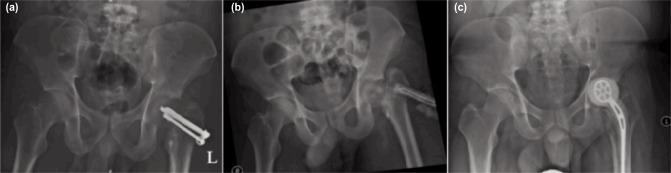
(a) On admission, radiograph showed femoral osteolysis surrounding the femoral neck screws with osteomyelitic changes of the femoral head, (b) Radiograph taken immediately after removal of implant(ROI) and insertion of antibiotic rods. Femoral neck collapsed and shorten after ROI and (c) A week after initial debridement, antibiotic-laden left hip spacer with metallic skeleton was inserted.
